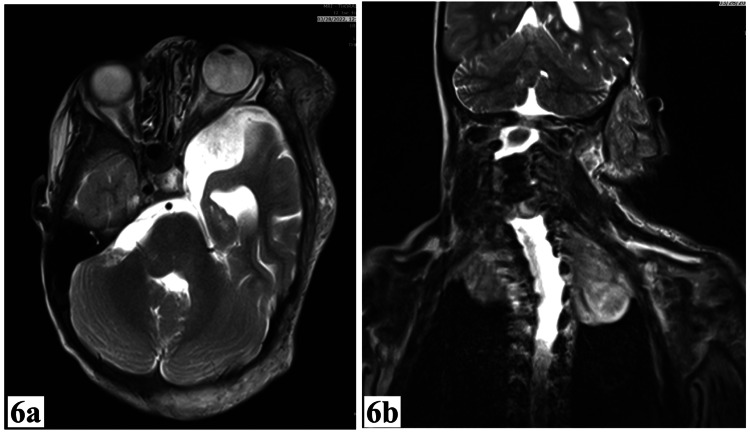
MRI of the patient. (a) Left eye proptosis and prominence of the left temporal lobe; (b) paravertebral masses are seen arising from the neural foramina and paravertebral regions.
